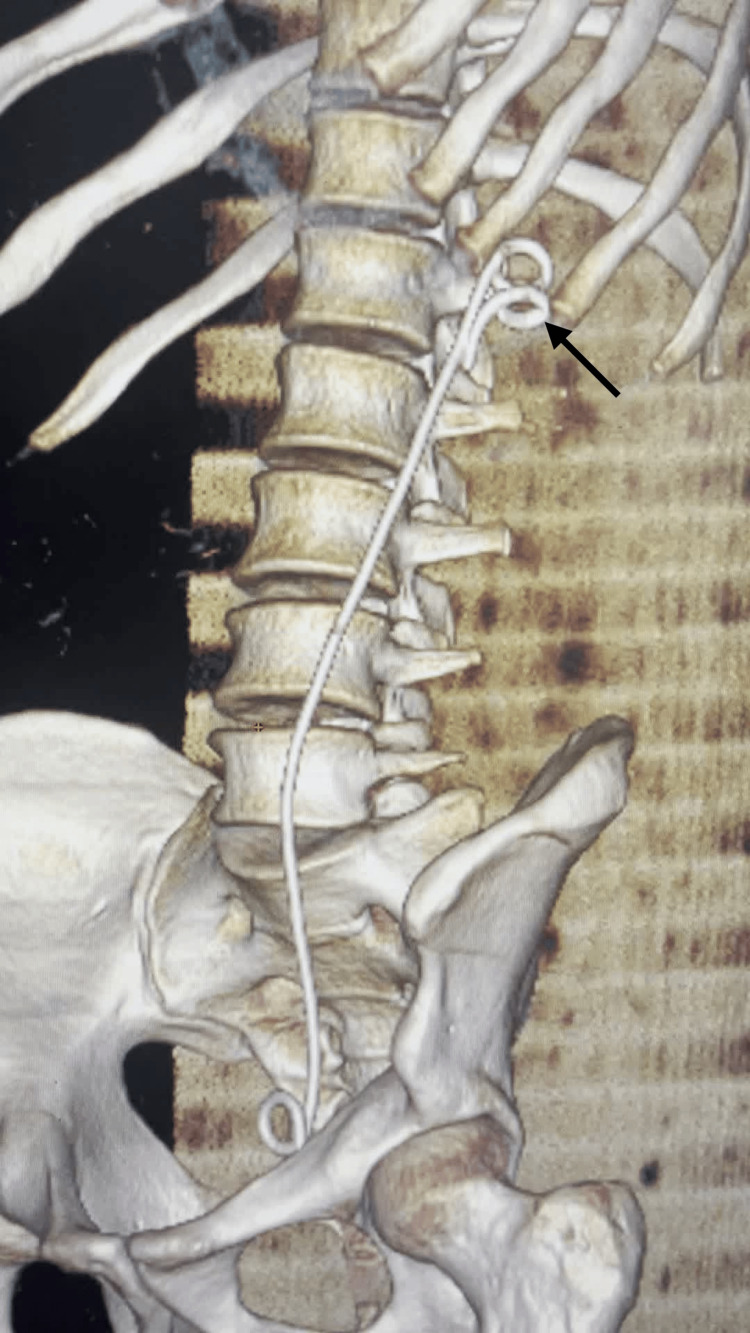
CT reconstruction showing a proximal loop of double-J stent (black arrow) abandoned into the renal parenchyma The proximal loop of the double-J stent was found to be embedded within the renal parenchyma during endoscopic exploration. The mobile portion of the stent was sectioned using a laser. Follow-up CT imaging showed the proximal loop remained in place, totally embedded within the renal parenchyma, while urinary drainage was maintained by the insertion of a new stent.
